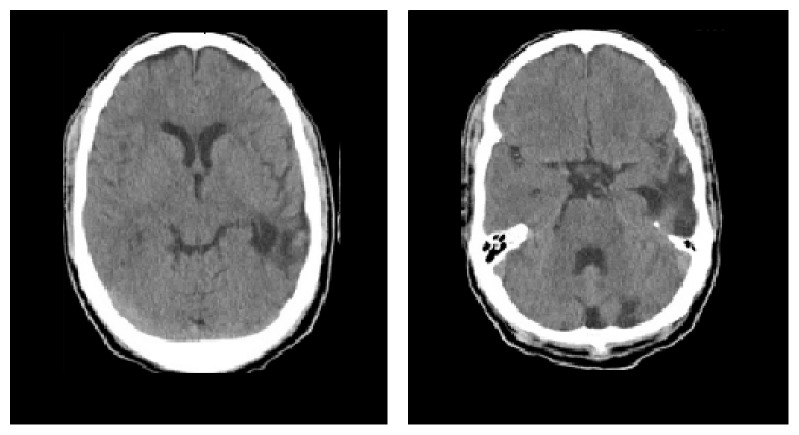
(Day 1) noncontrast CT head showing encephalomalacia on the left hemisphere without hemorrhage.
